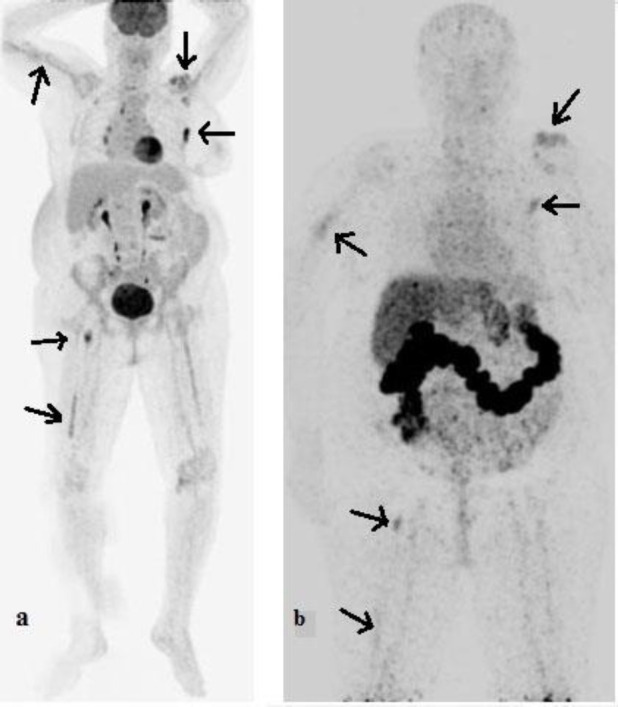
PET/CT images of a woman with HER2-positive metastatic breast cancer after injection of [^18^F]FDG (a) and [^89^Zr]Zr-Trastuzumab [96 h (b)]
